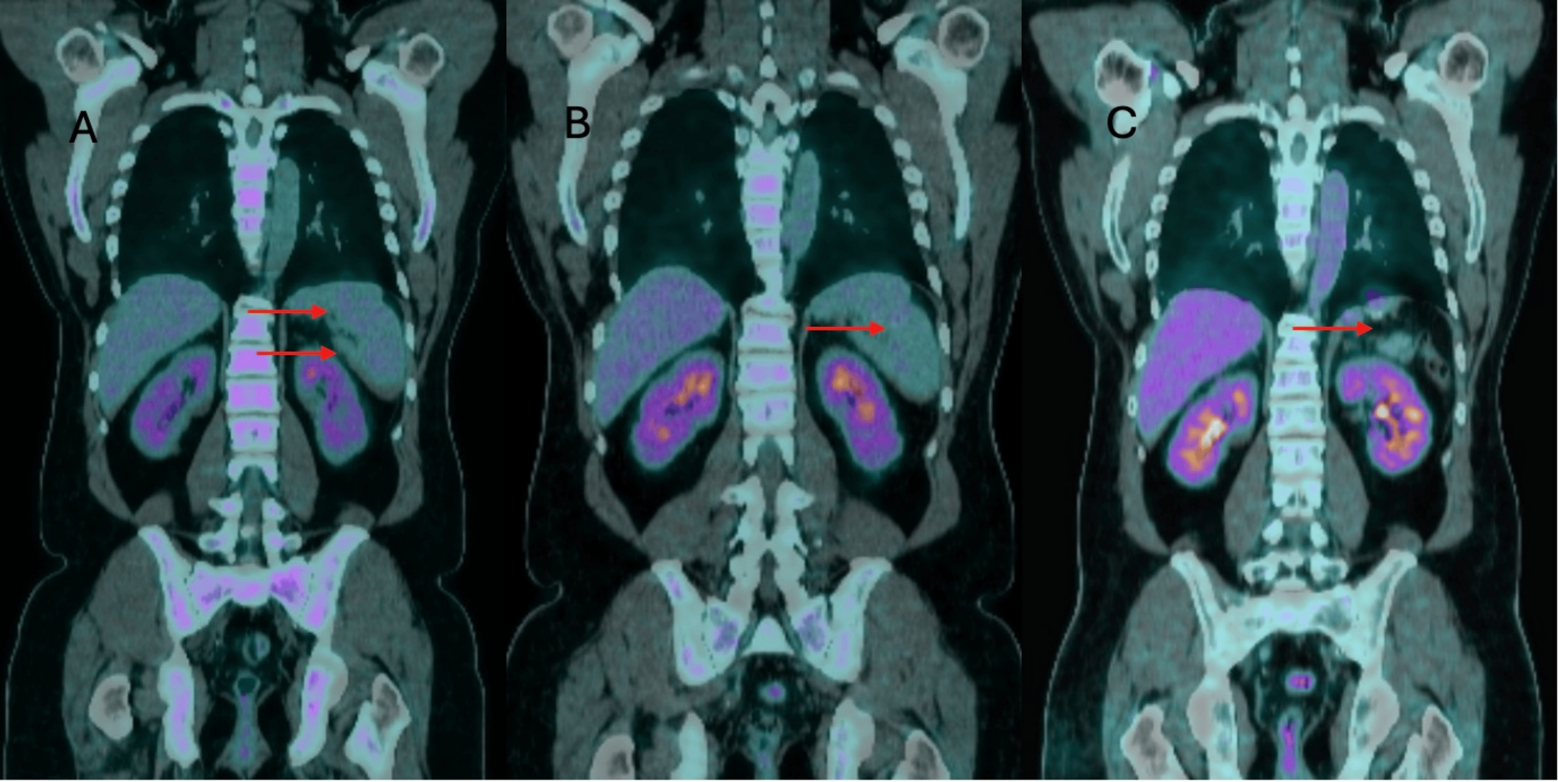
FDG PET/CT response assessment after cycle 4 and 6 after chemo-immunotherapy followed by metabolic resolution post-splenectomy. A (left): PET/CT following cycle 4 of chemo-immunotherapy showing partial metabolic response with residual uptake in enlarged spleen (red arrows). B (middle): PET/CT after completion of cycle 6 demonstrating further reduction in splenic metabolic activity and volume with persistent fludeoxyglucose (FDG) uptake (red arrow). C (right): PET/CT showing complete metabolic response post-splenectomy (red arrow indicates the anatomical site of removed spleen).
